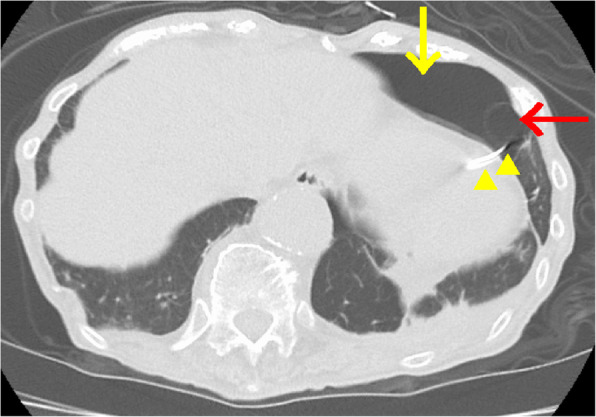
A preoperative chest computed tomography image. Thoracic computed tomography showing a left-sided pneumothorax (yellow arrow) and the pacemaker lead (yellow arrowheads) perforating the right ventricle and lung tissue neighboring a pulmonary cyst (red arrow)
